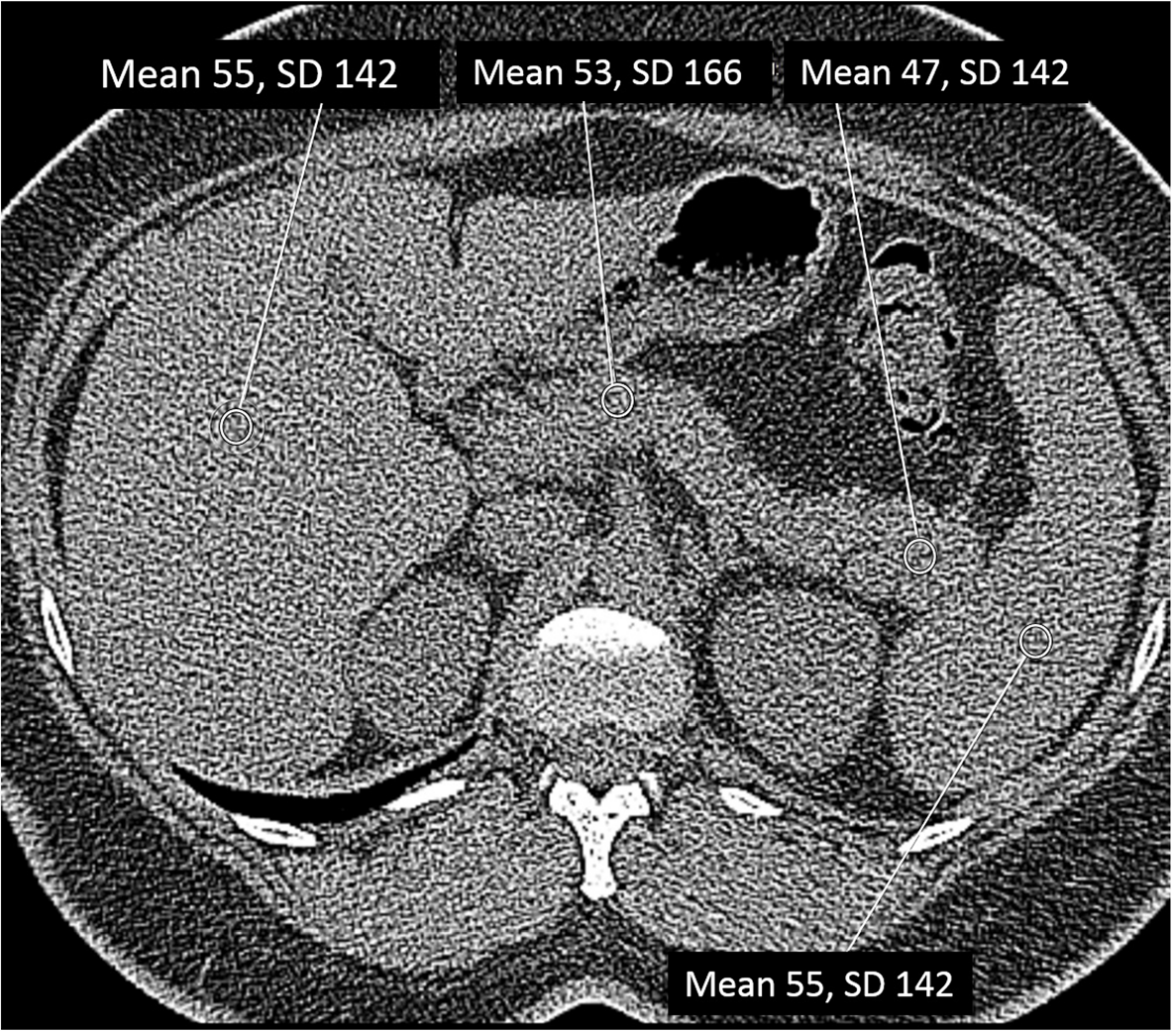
HRCT of the chest including the upper abdomen. The image shows from left to right a region of interest for measurement of the CT number (Hounsfield units) placed in the liver, pancreatic body and tail, and spleen respectively. The measurements of the spleen were used for normalization of attenuation (Hounsfield units) to compensate for variability between different CT machines
